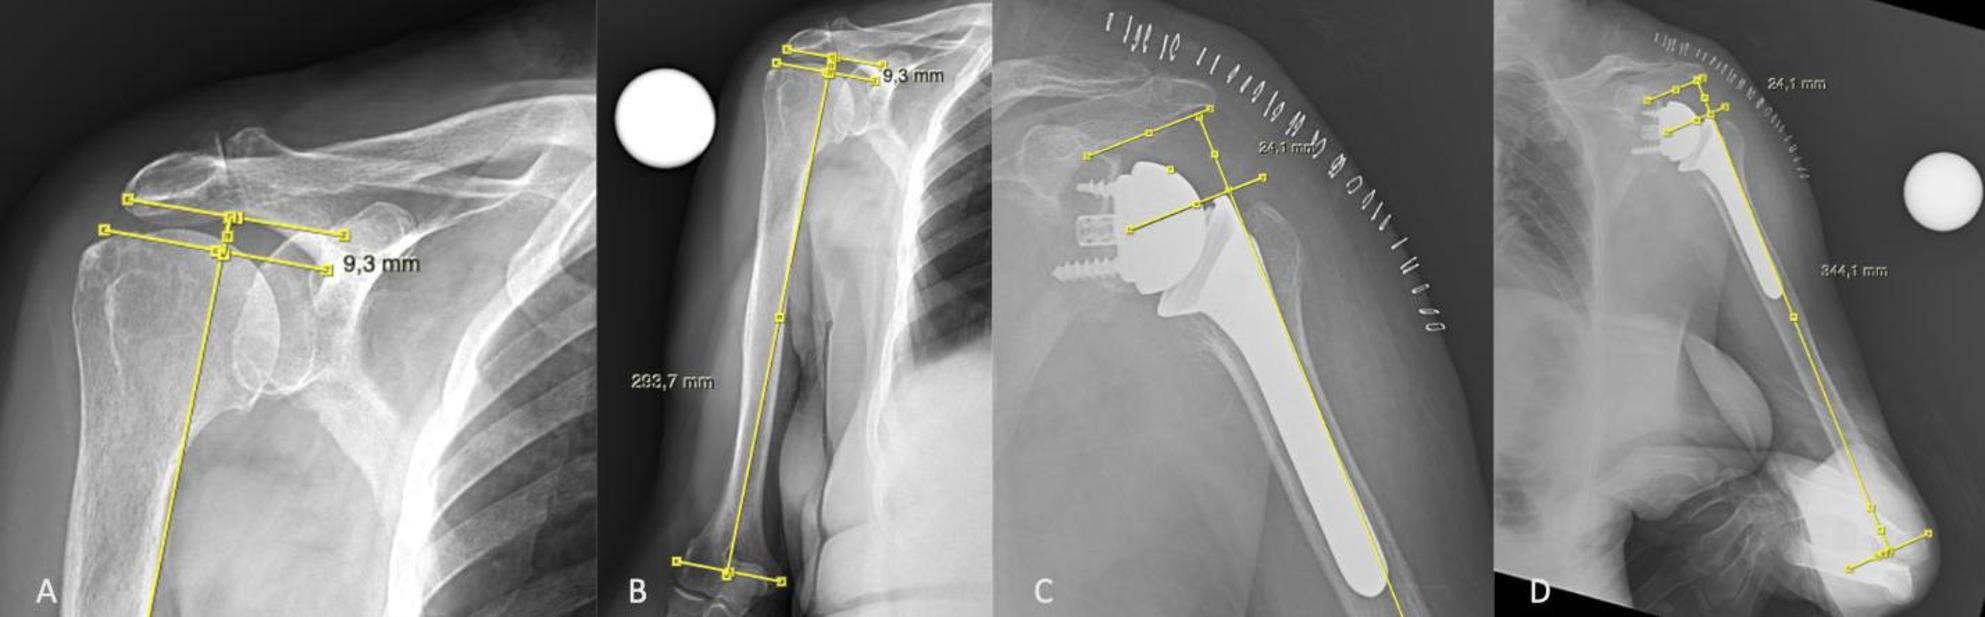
A, preoperative extremity length measurement on AP x-ray; B, preoperative acromiohumeral distance measurement on AP x-ray; C, postoperative extremity length measurement on AP x-ray; 1D, postoperative acromiohumeral distance measurement on AP x-ray
